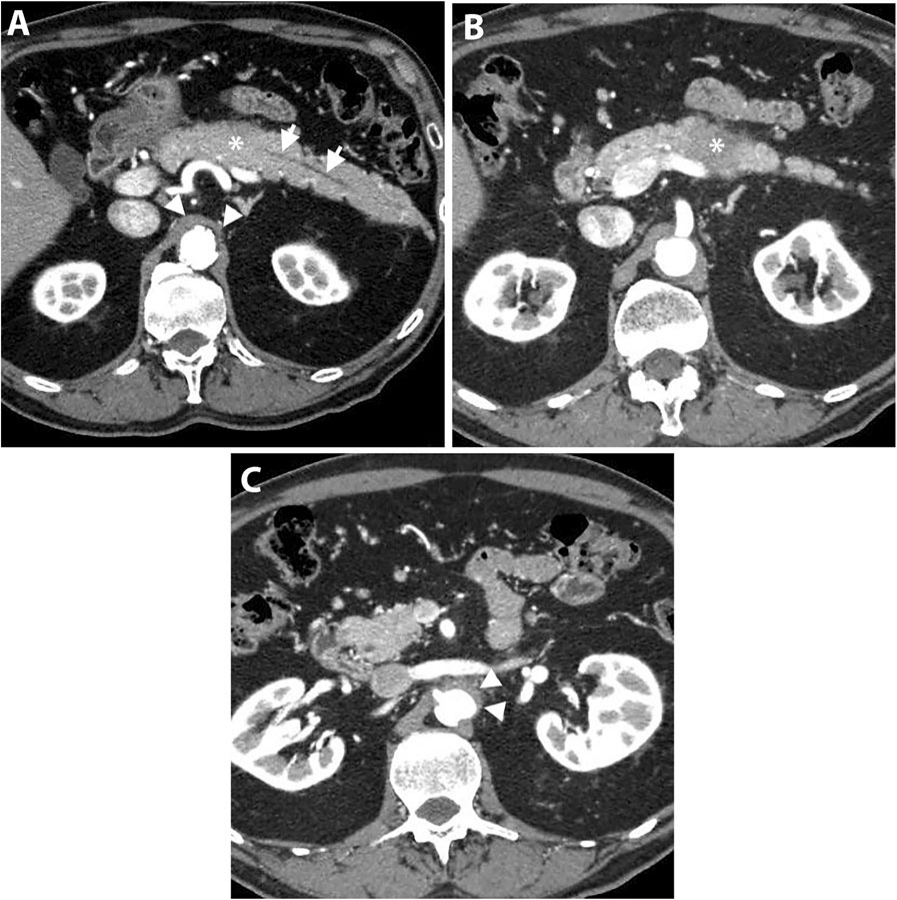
A 71-year-old man with a mass-forming AIP. Axial (a–c) pancreatic phase CT images show two ill-defined, mass-like lesions (asterisk) in the pancreatic body without significant MPD dilatation (arrows). There is associated rind of periaortic soft tissue thickening (arrowheads) representing IgG4-related retroperitoneal fibrosis
